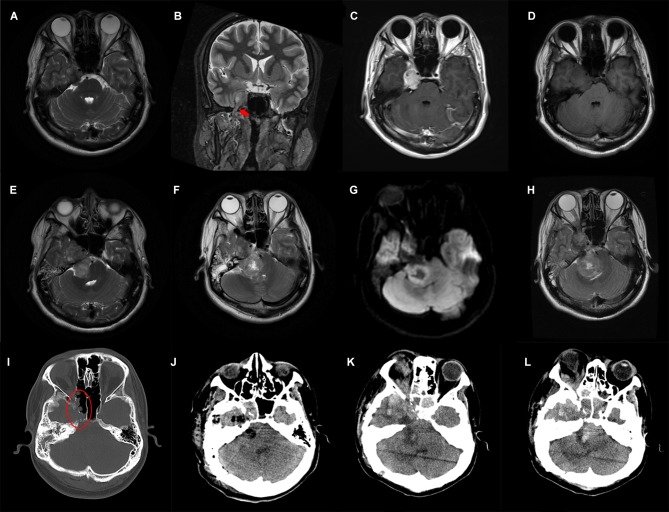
Preoperative and postoperative imaging features of the patient. T2-weighted MRI axial (A) and coronal (B) images show a solid tumor arising from the trigeminal nerve (red arrow) with heterogeneous contrast-enhanced (C); (D–H) Axial MRI images reveal gradual enlargement of the tumor; (I) Destruction of the adjacent skull base bone is shown in the oval; (J) Postoperative CT shows subtotal removal of the tumor; (K,L) Follow-up CT scanned 3 weeks after surgery shows tumor recurrence.
